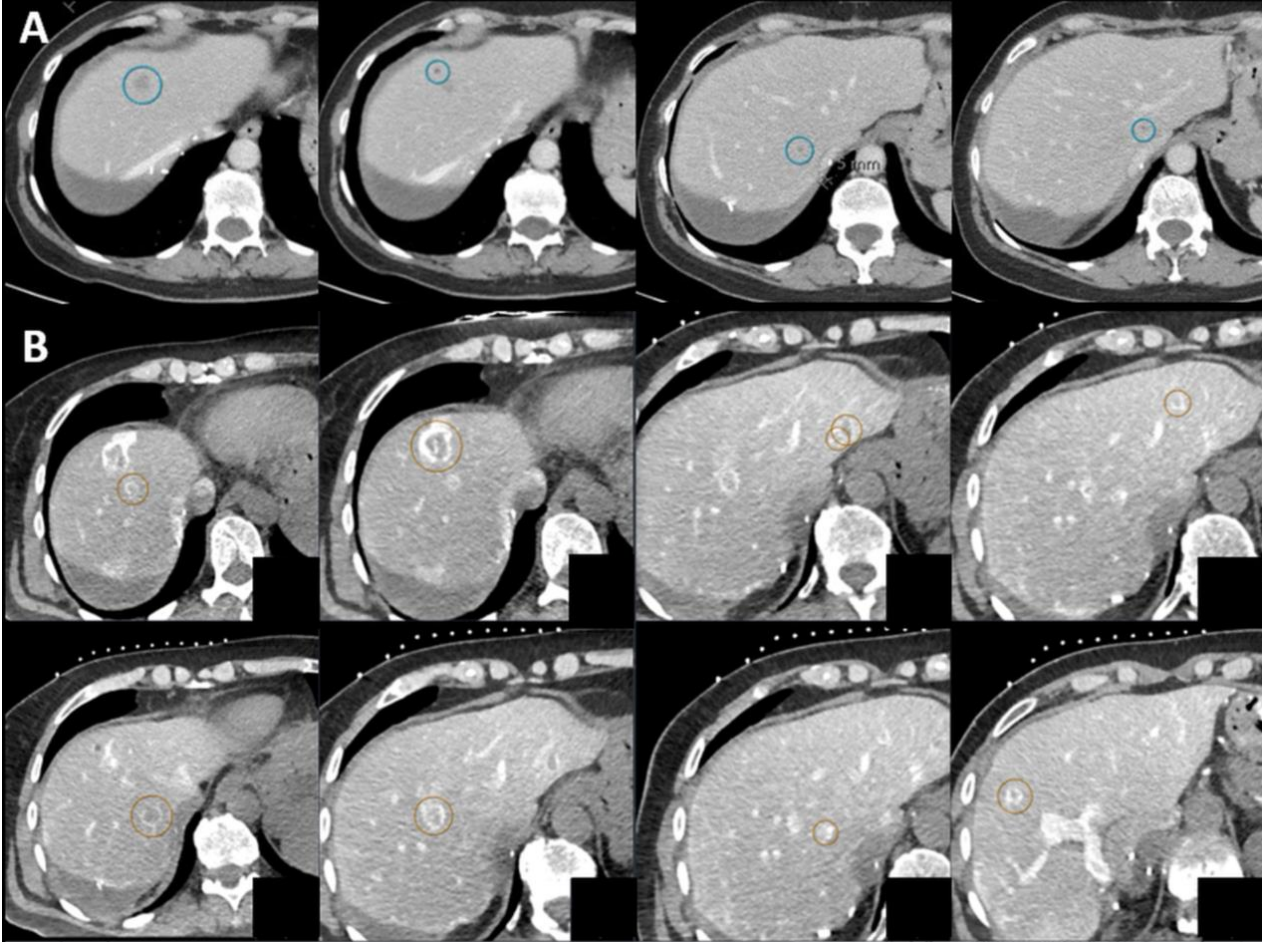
Detection of additional lesions during the procedure. Progressive disease after partial right-sided hepatectomy manifesting in four tumors (blue circle) seen on follow-up contrast-enhanced CT (A). The percutaneous microwave procedure was planned within three weeks after the follow-up scan. During the procedure, after catheterization of the proximal common hepatic artery administration of 40 mL Xenetix 300^®^ (1:1 mixed bolus of saline and contrast agent; Iobitridol 300 mg Iodine/mL, Guerbet, Villepinte, France), at least five additional ring-enhancing lesions (red circles) were found throughout the liver (B). Due to the extensiveness of the disease, the procedure was terminated. One day after the procedure, the additional lesions were confirmed to be metastases showing diffusion restriction on MRI.
